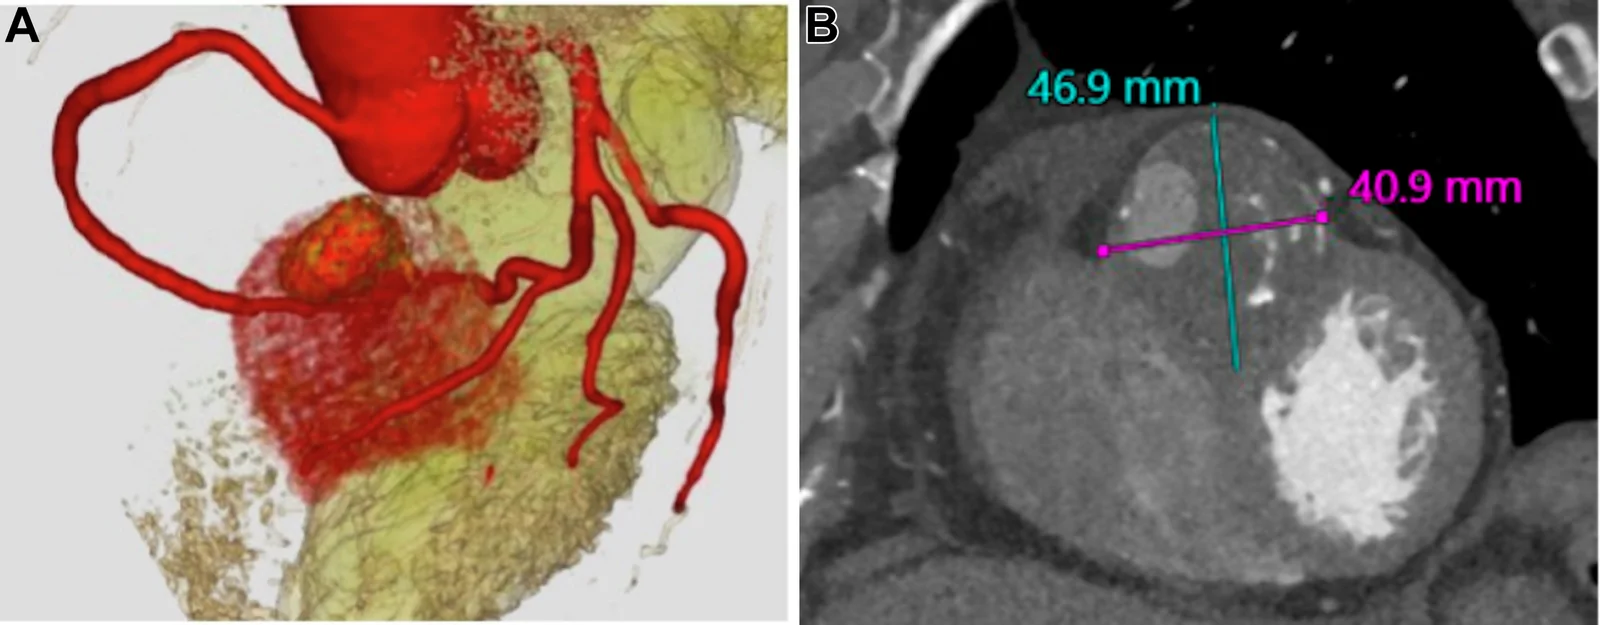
Coronary Computed Tomography Assessment of Coronary Aneurysm Coronary computed tomography showing a three-dimensional rendering (A) and sagittal view (B) of a massive (47 × 41 mm), circular aneurysmal mass, supplied by a large septal branch.
